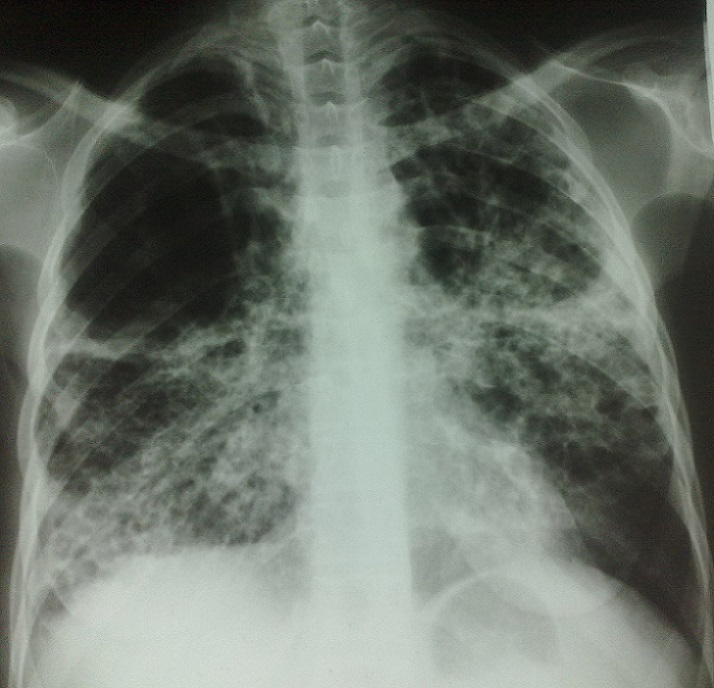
Radiographie du thorax, Tuberculose pulmonaire étendue et bilatérale chez une femme
